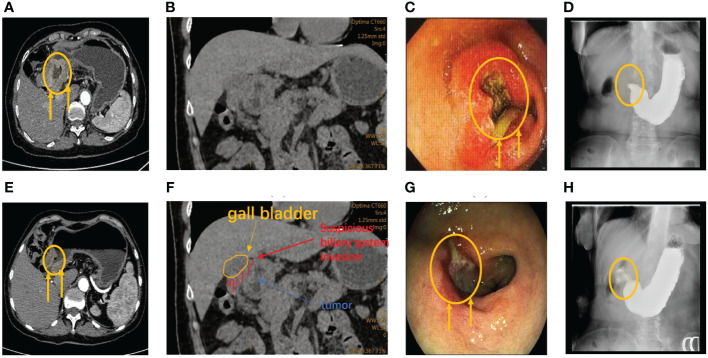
(A) CT reveals abnormal thickening of the antrum wall and incomplete obstruction prior to therapy. (E) Following three treatment cycles, CT scans showed significant improvement with disease regression, tumor retraction, and alleviated obstruction. (B, F) CT examination after admission showed suspicious biliary invasion. (C) Before therapy, gastroscopy revealed nearly complete stenosis of the pyloric ring cavity, accompanied by obstructive and edematous surrounding tissues. (G) Preoperative gastroscopy revealed obvious tumor retraction, along with significantly reduced obstruction and tissue edema compared to pretherapy gastroscopy. (D, H) Barium meal shows incomplete pyloric obstruction before the second cycle of treatment.
